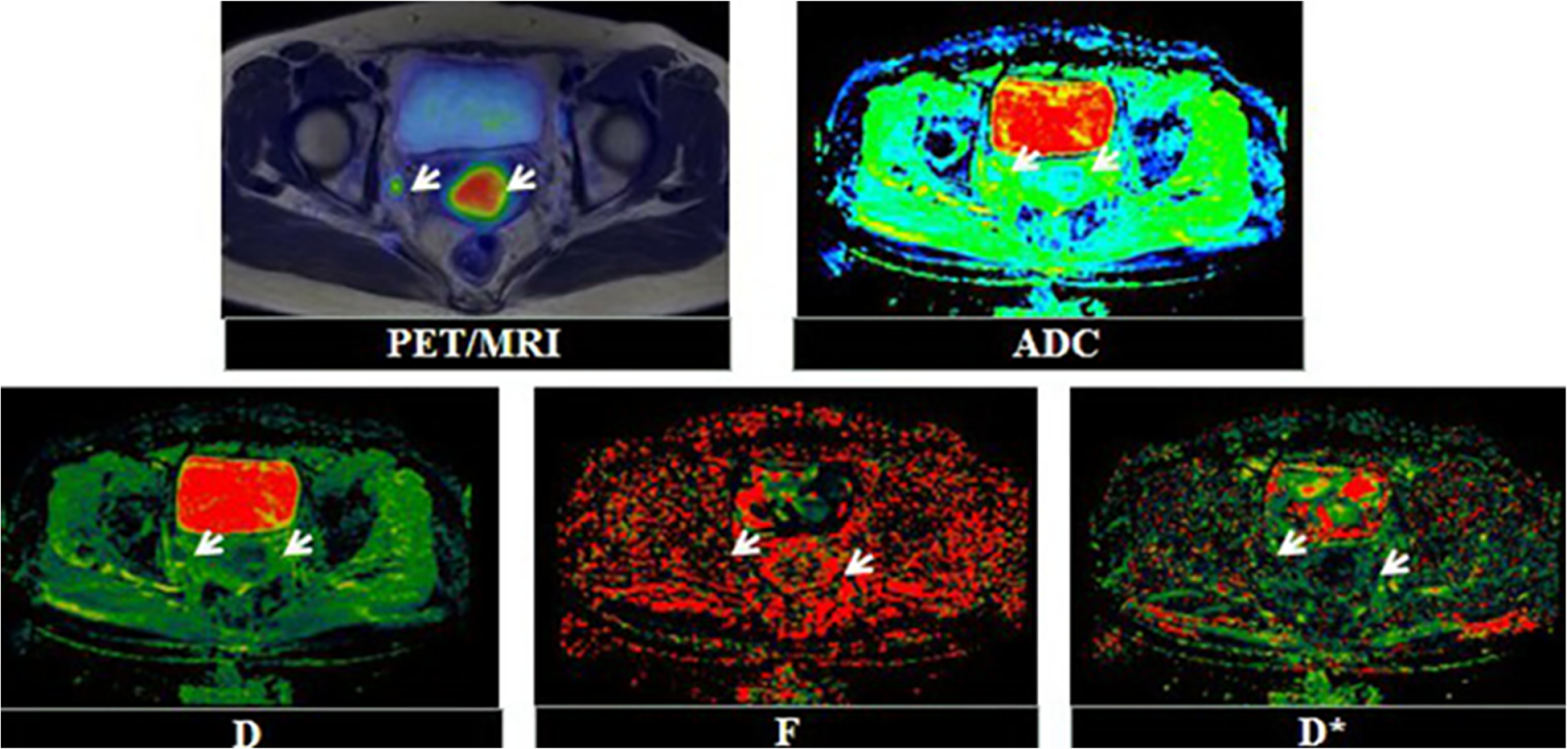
Forty-nine years-old female patient diagnosed with poorly differentiated squamous cervical carcinoma with a suspected lymph node metastasis showed on preoperative PET/MR. Postoperative pathology confirmed it as a metastatic lymph node. PET and PET/MR fusion images showed that FDG uptake in the tumor (SUV_max_ = 14.08 g/cm^3^, SUV_mean_ = 9.35 g/cm^3^, TLG = 326.69 g, MTV = 34.94 cm^3)^, and the ADC and IVIM image showed a high signal in the lymph node (ADC_mean_ = 1.27 × 10^− 3^ mm^2^/s, ADC_min_ = 0.67 × 10^− 3^ mm^2^/s, D_mean_ = 0.97 × 10^− 3^ mm^2^/s, D_min_ = 0.51 × 10^− 3^ mm^2^/s, D* = 35.92 × 10^− 3^ mm^2^/s, f = 0.17)
